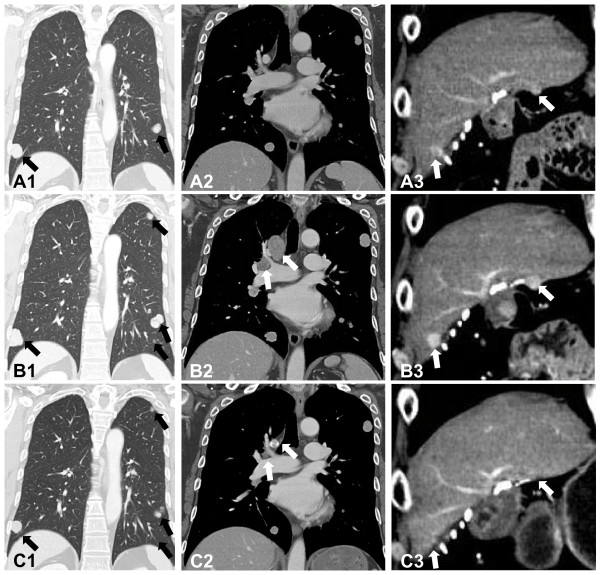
(A1-3) Contrast-enhanced computed tomography scans before restarting sorafenib. (A1, 2) Multiple metastatic lesions were observed in the lung (black arrows). (A3) Local tumor progressions were also observed in the cut surface of the liver (white arrows). (B1-3) Contrast-enhanced computed tomography scans six months after restarting sorafenib. (B1) The growth and new metastatic lesions were observed in the lung (black arrows). (B2) In addition, mediastinal lymph node metastases were observed anew (white arrows). (B3) Local tumor progressions were unequivocally observed (white arrows). (C1-3) Contrast-enhanced computed tomography scans 10 months after restarting sorafenib. (C1) Metastatic lesions in the lung shrunk or disappeared (black arrows). (C2-3) Mediastinal lymph node metastases and local tumor progressions completely disappeared (white arrows).
